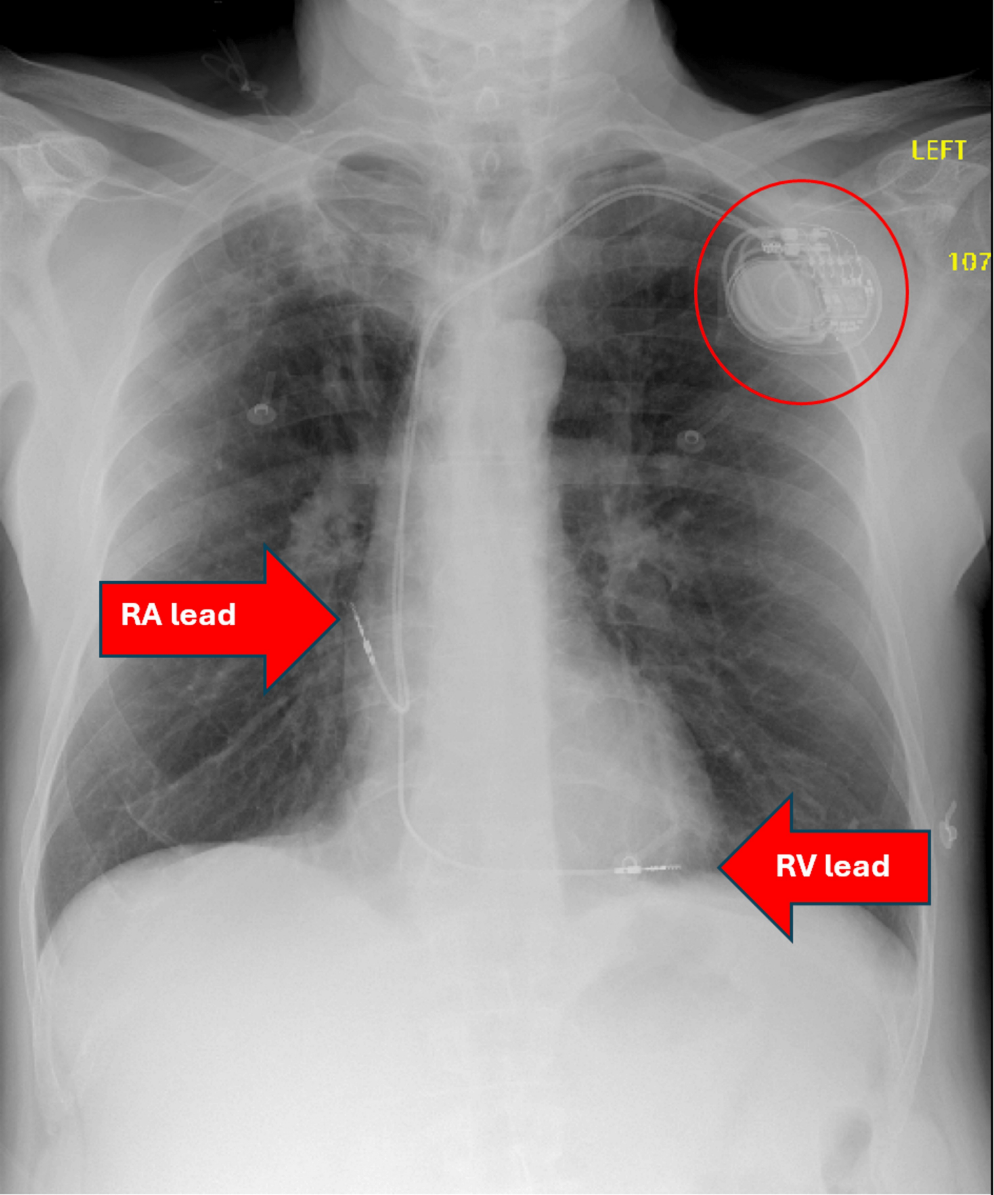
Initial Chest X-Ray Plain chest radiography showing pacemaker device with distal lead tips seemingly in appropriate position within the right atrium (RA) and right ventricle (RV). No obvious pericardial effusion or focal consolidation noted.
